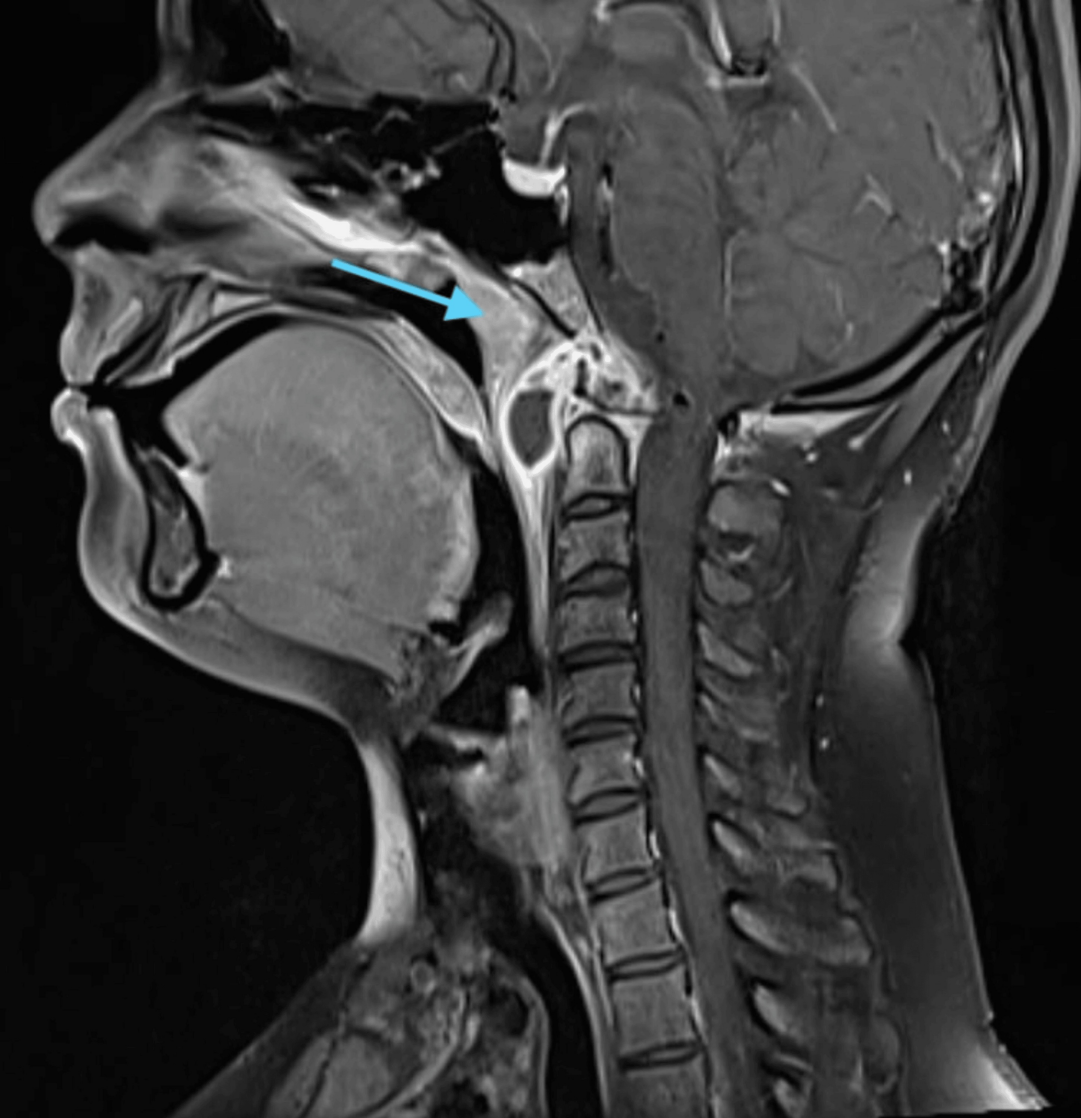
MRI cranio-vertebral junction - sagittal plane Altered marrow signals are noted in the clivus, anterior arch of the C1 vertebra, its right lateral mass, adjoining posterior neural arch, dens, and adjoining body of the C2 vertebra, which appear hypointense on T1WI. Erosion and destruction are noted involving the clivus (blue arrow).
